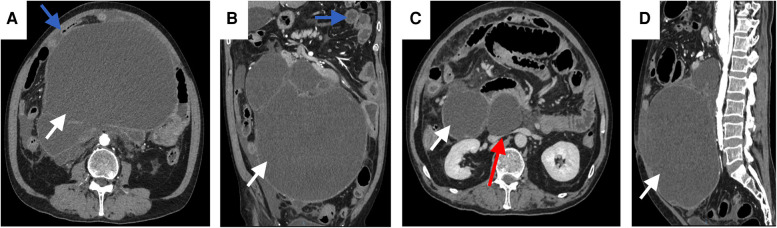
Abdominal enhanced CT axial arterial phase (A), coronary arterial phase (B), axial venous phase (C),and sagittal arterial phase (D) CT images show a retroperitoneal cystic mass (white solid arrow), extending along the mesentery to 24 cm. The tumor oppresses the surrounding intestine (blue solid arrow) and the inferior vena cava (B) (red solid arrow).
